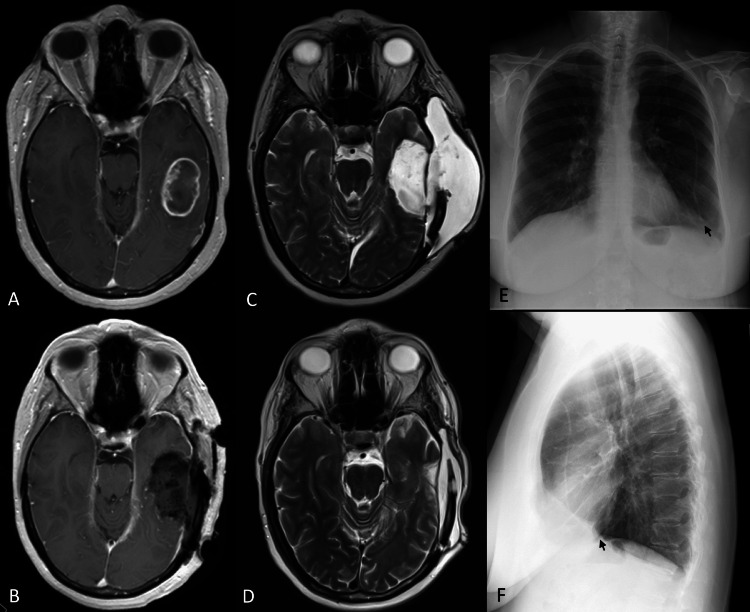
Pseudomeningocele resolved without compressive head dressing (A) Pre-operative gadolinium-enhanced T1-weighted magnetic resonance imaging (MRI) of the brain revealed a ring-enhancing mass at the left temporal lobe consistent with a neoplasm. (B) Post-operative gadolinium-enhanced T1-weighted MRI revealed a gross total resection of the lesion (pathology: World Health Organization grade IV glioma). (C) T2-weighted MRI revealed a sizeable pseudomeningocele. (D) T2-weighted MRI obtained following subgaleal-pleural shunt placement revealed resolution of the pseudomeningocele. (E, F) Anteroposterior and lateral view chest X-rays demonstrating the distal end of the catheter ending in the left pleural space (black arrow).
